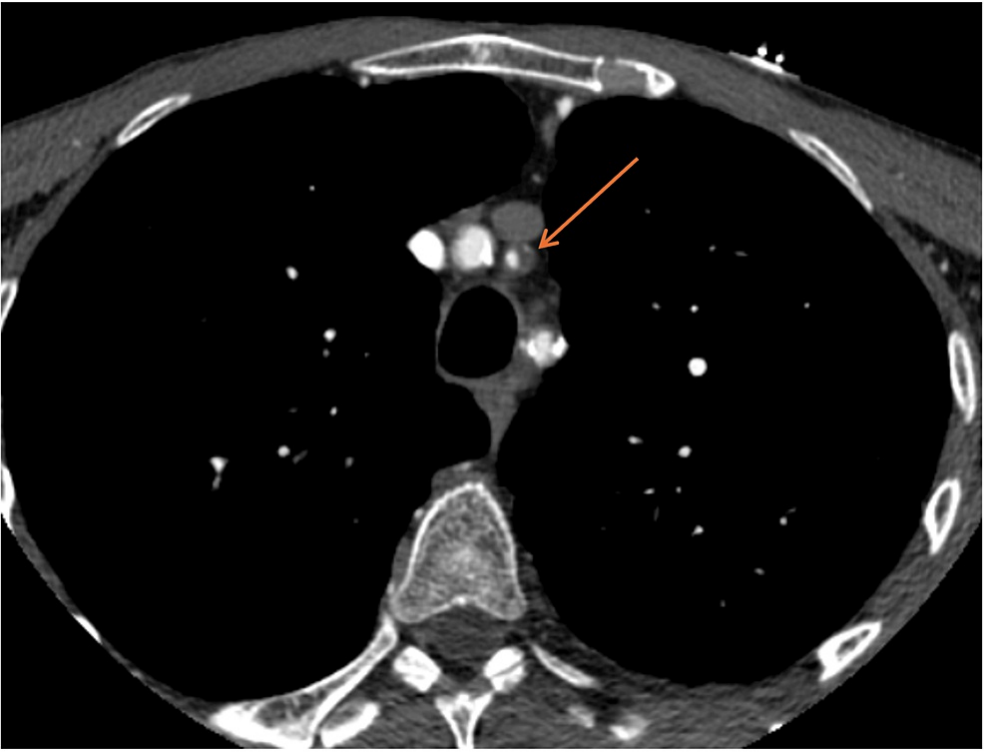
Axial view of a CT angiogram depicting in-stent restenosis of left common carotid artery stent (arrow).
